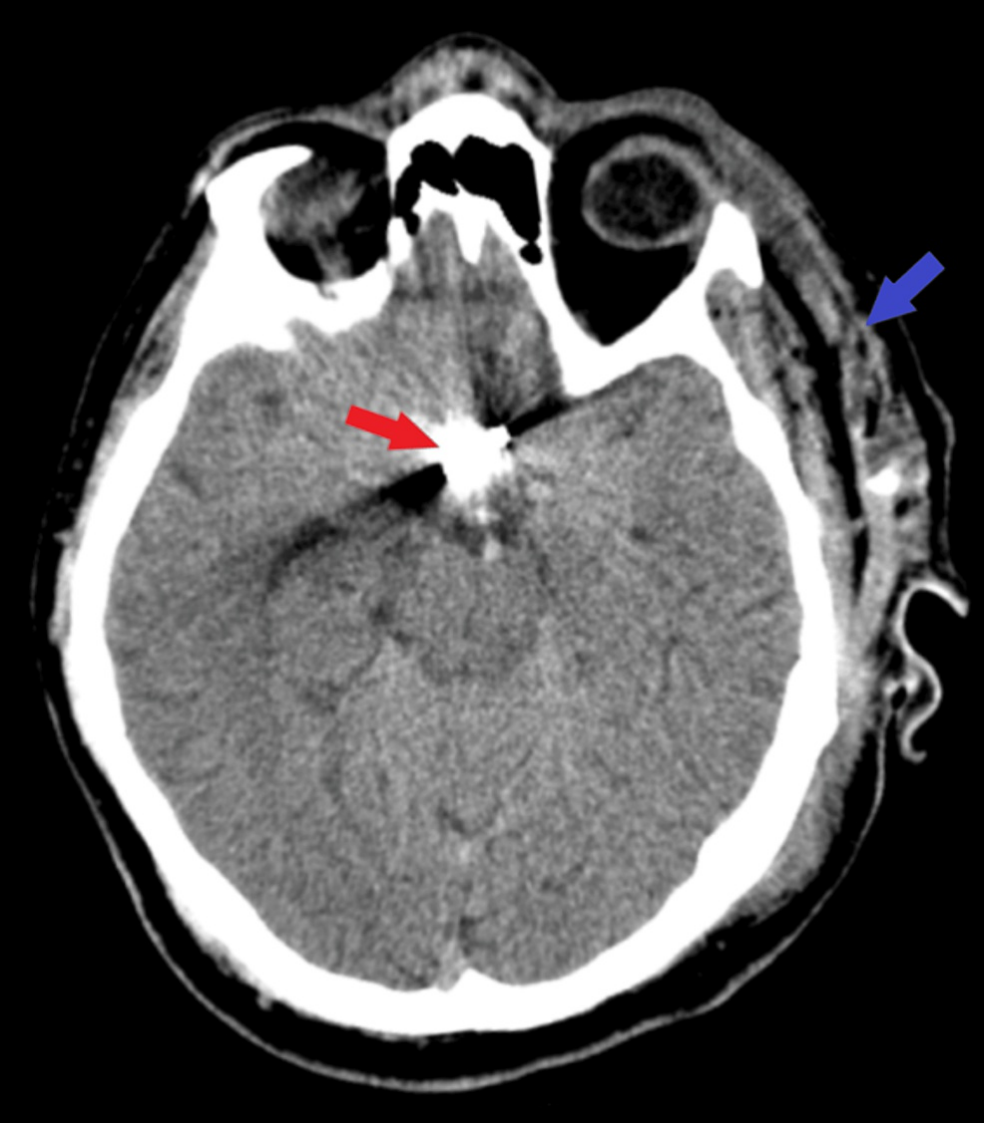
Postoperative CT scan. The axial section of tissue window CT examination demonstrates the proper clip positioning (red arrow), along with successful aneurysm clipping. A dehiscence lesion was observed on the left side (blue arrow) which was subsequently remitted.
